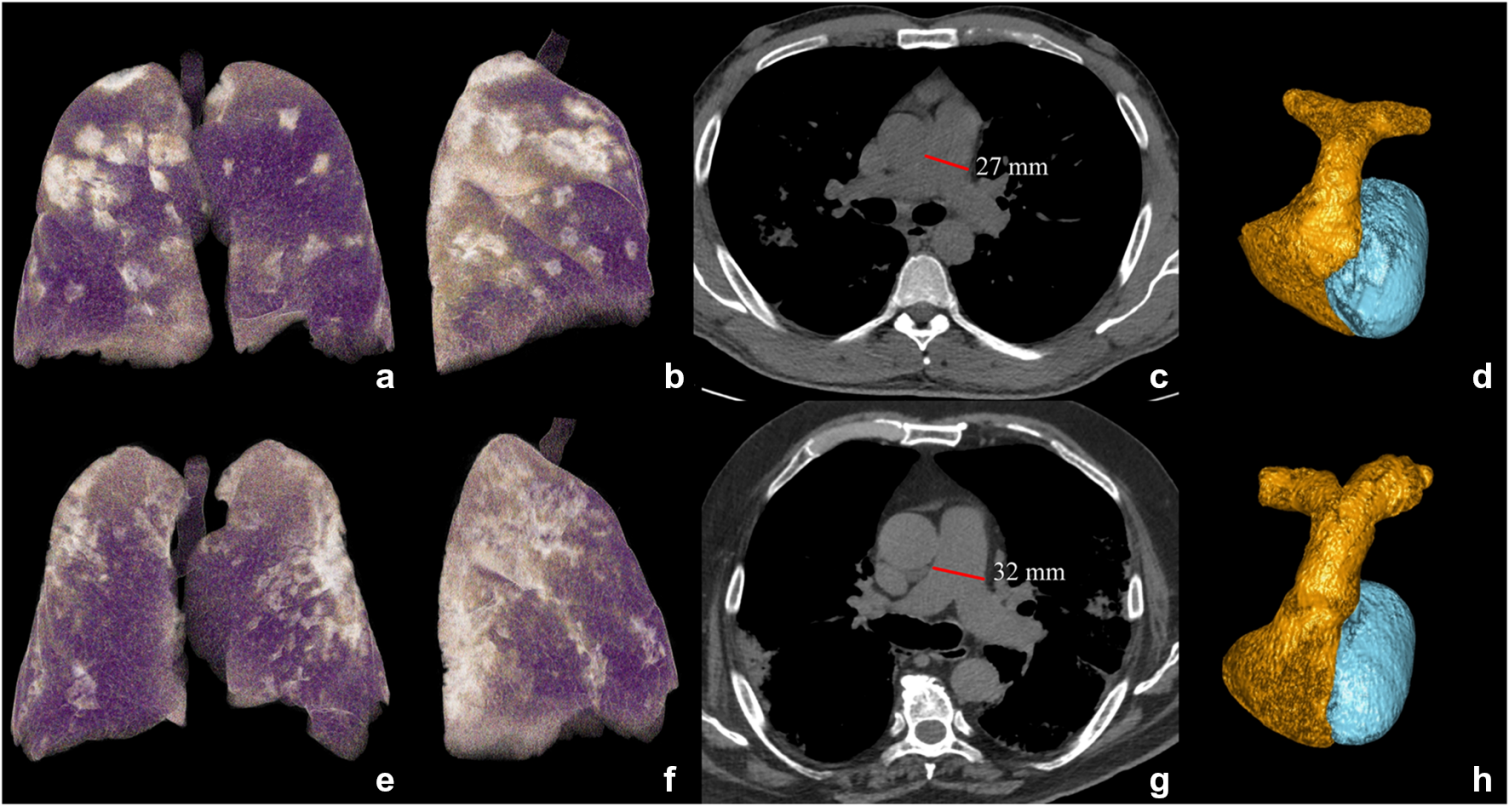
Exemplifying cases: a survivor (top) and non-survivor (bottom) patients. CT 3D volume rendering of lungs in antero-posterior view (a, e) and lateral view (b, f), CT axial images with mediastinal window (c, g) for main pulmonary artery diameter (MPAD) measurement (red lines in c, g), and 3D volumetric reconstruction of pulmonary arteries (d, h) in a survivor (top) and a non-survivor (bottom) COVID-19 patient, both males of 74 and 76 years, respectively, both with history of hypertension and treated ischemic cardiomyopathy, both suffering from fever (> 37.5 °C) and caught from 7 days. At the admission, WBC/mm^3^ were 4.36 and 3.8, CRP 0.93 and 1.1 mg/dL, LDH 295 and 378 U/L, and creatinine 0.93 and 1.1 mg/dL in survivor and non-survivor, respectively. The oxygen saturation was 90% and 94% for survivor and non-survivor, respectively, with higher well-aerated lung volume in the survivor patient (3882 mL vs 2382 mL, violet parenchyma on 3D lung volume rendering in a, b, e, f) with pneumonia (bright parenchyma in a, b, e, f), involving from 25 to 50% of lung volume in both cases. Pulmonary artery diameter was normal (27 mm) in the survivor (c, d) while it was enlarged (32 mm) in the non-survivor (g, h) who died 9 days after hospital admission
